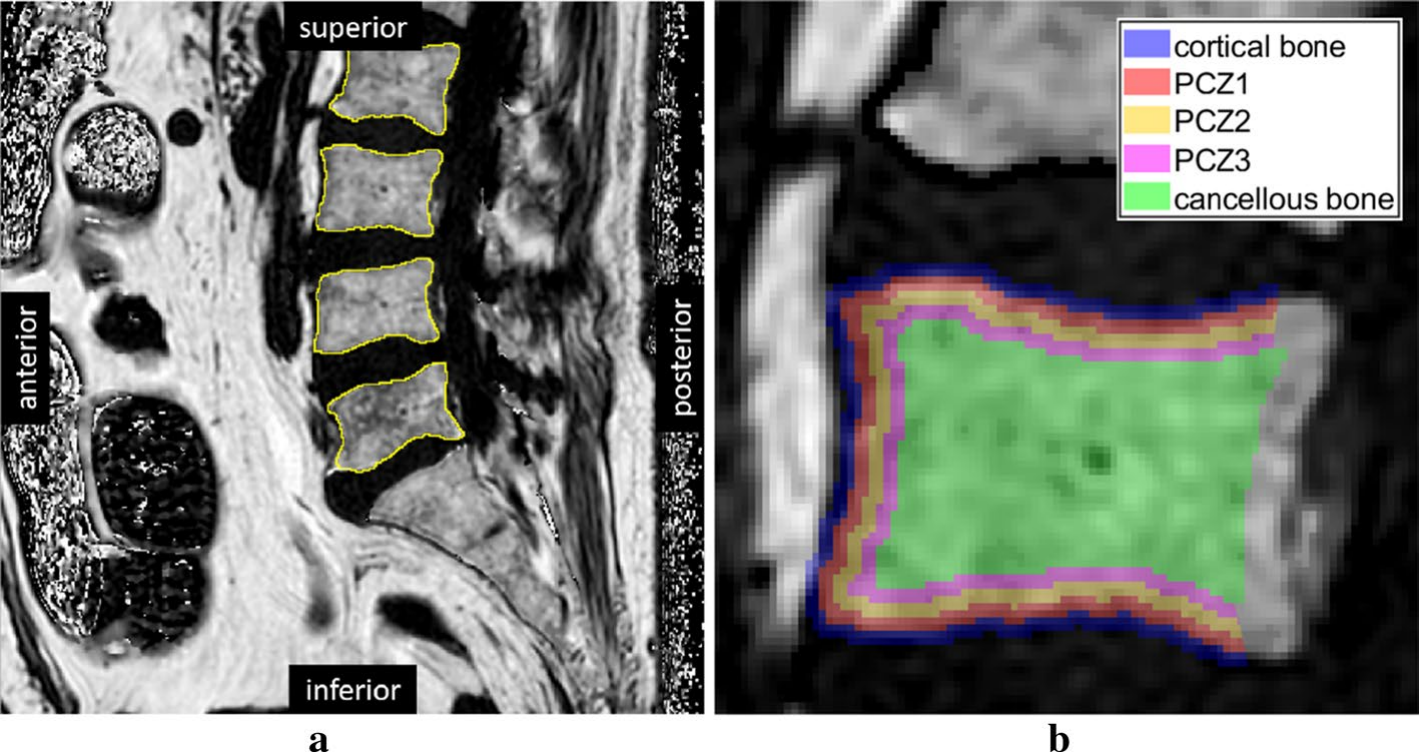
ROI selection process: a Mid-sagittal PDFF MR image of the lumbar spine with detected vertebra contours b Definition of the anatomical ROIs: cortical bone, pre-cortical zone (PCZ), and cancellous bone
